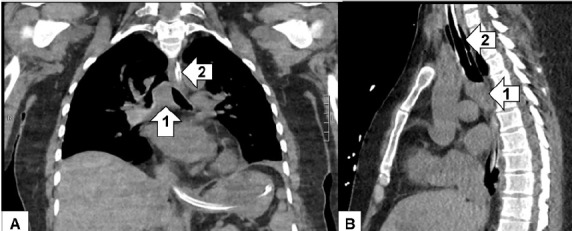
Coronal (A) and sagittal (B) computed tomography of the chest without contrast of a 40-year-old female with dyspnea showing a mass at the carina (1). (Trachea with endotracheal tube [2] is marked to orient the reader.)
